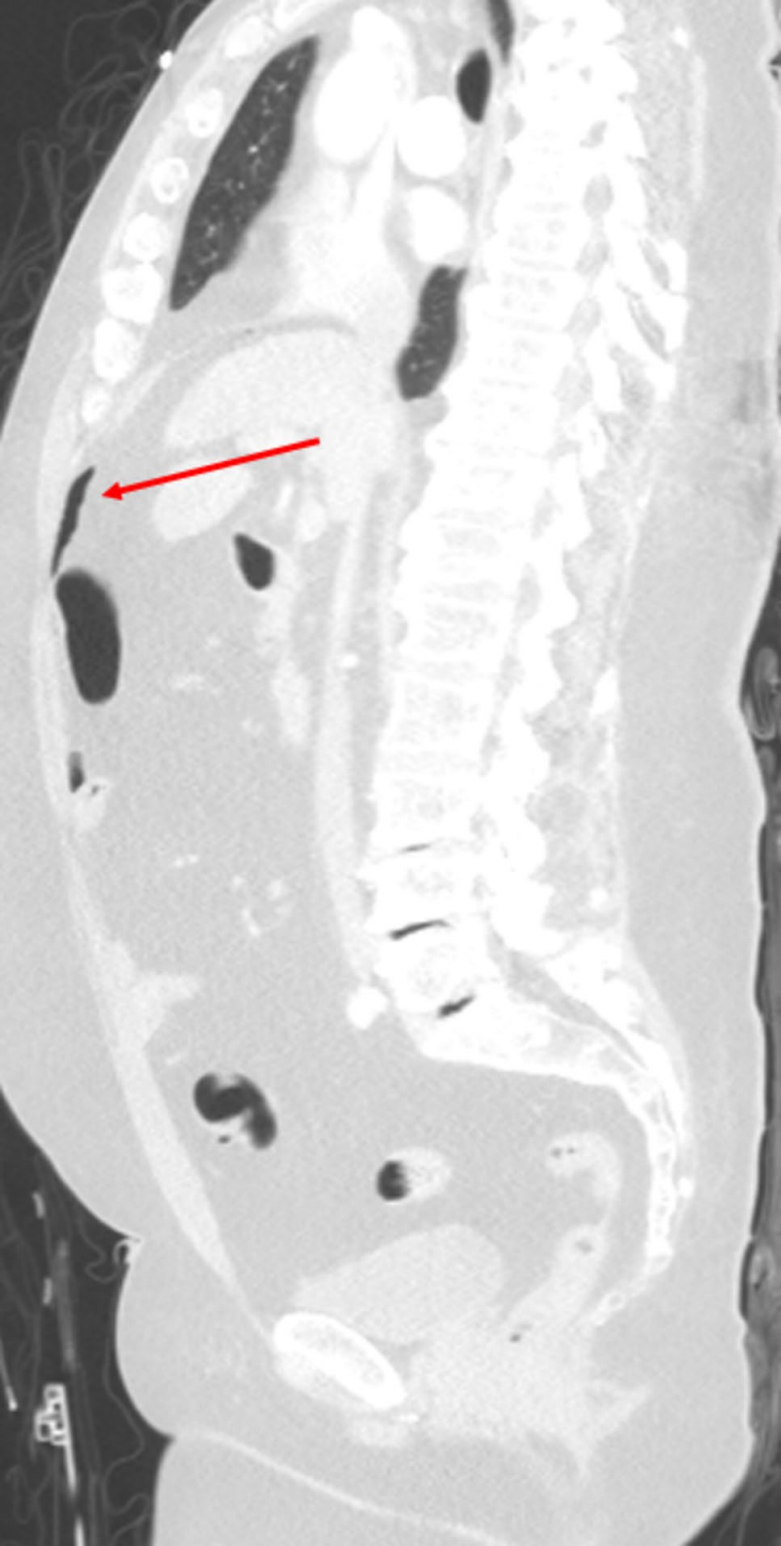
Sagittal CT in lung window demonstrates a large amount of free air (red arrow). There were no other signs of bowel injury according to the RAPTOR grading; however, the patient was confirmed to have an injury of the distal ileum. This was graded as a 2 given the large volume of free air
